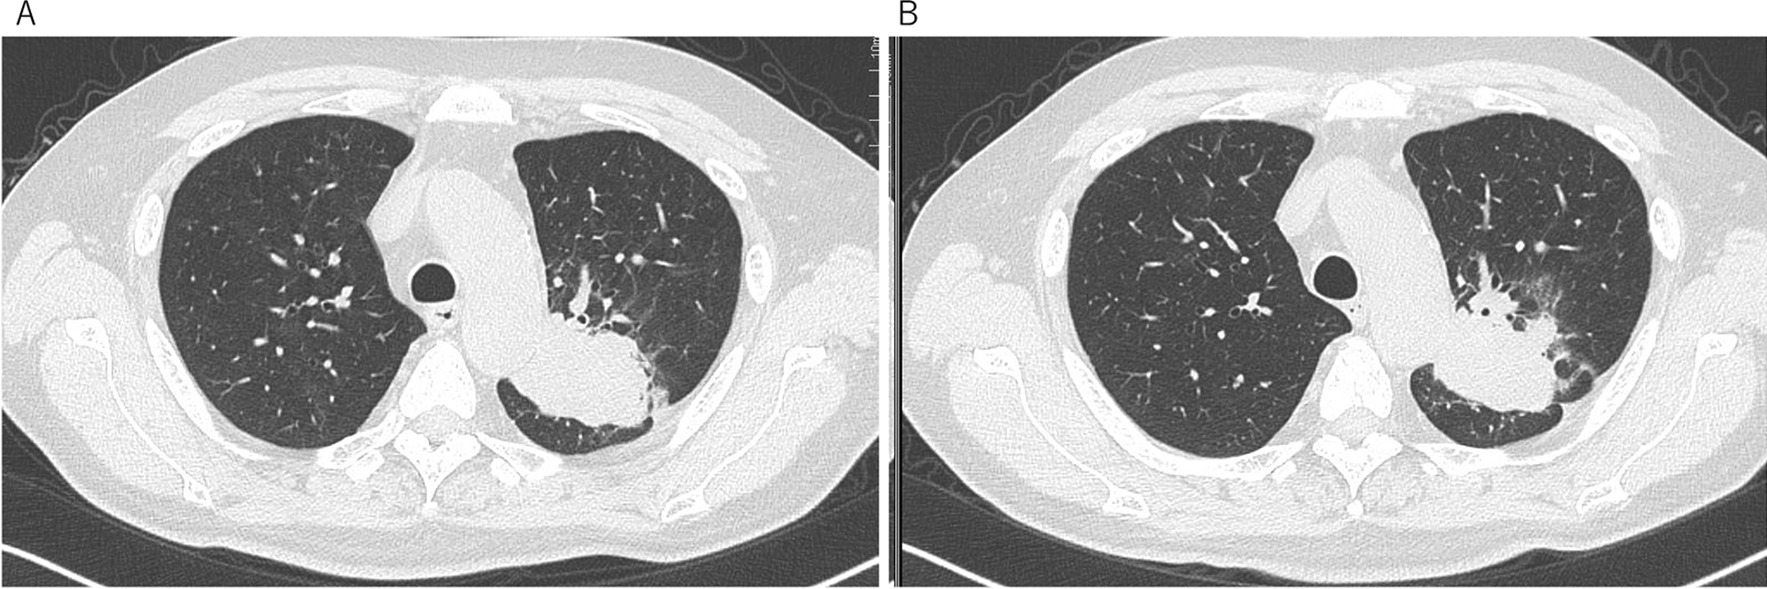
Thin-slice CT before and after the administration of lorlatinib. Compared with the patient’s condition before the administration of lorlatinib (a), SD has been maintained 33 months after the start of lorlatinib (b), and no recurrence of DILD has been observed. *CT* computed tomography, *SD* stable disease, *DILD* drug-induced interstitial lung disease
